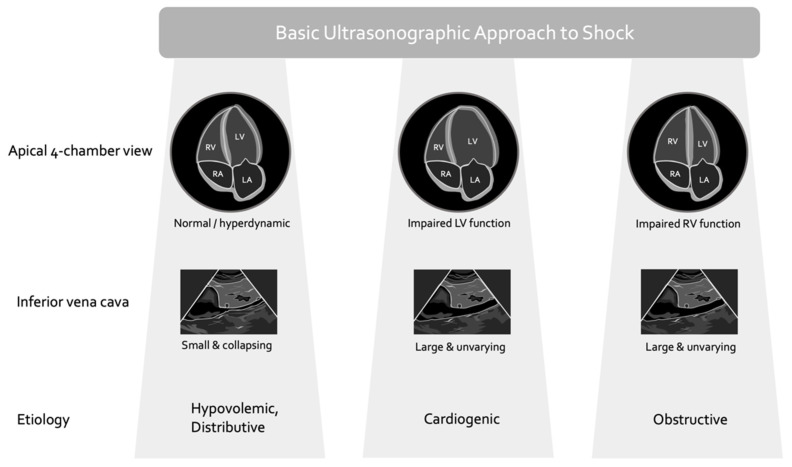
Basic ultrasonographic approach to shock. RA and LA are right and left atria; RV and LV are right and left ventricles. These are simplifications, obstructive shock may be due to pericardial effusion or tension pneumothorax in addition to pulmonary emboli or severe acute respiratory distress syndrome (ARDS). Additionally, shock is often multifactorial; e.g., a patient with septic shock from pneumonia complicated by severe ARDS may have all 4 etiologies occurring in concert.
